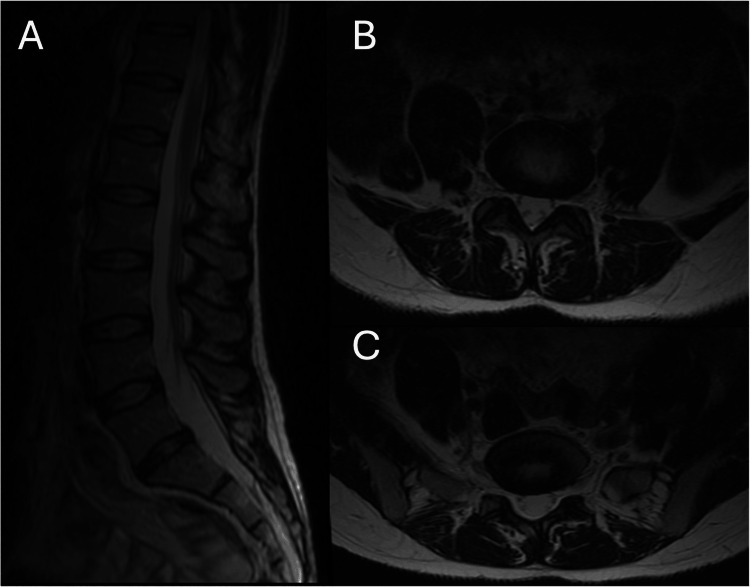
Lumbar MRI. Sagittal (A) and axial L4-L5 (B) and L5-S1 (C) MRI showed a bulging disc at L4-L5 and L5-S1 levels and no evidence of nerve root compression.
